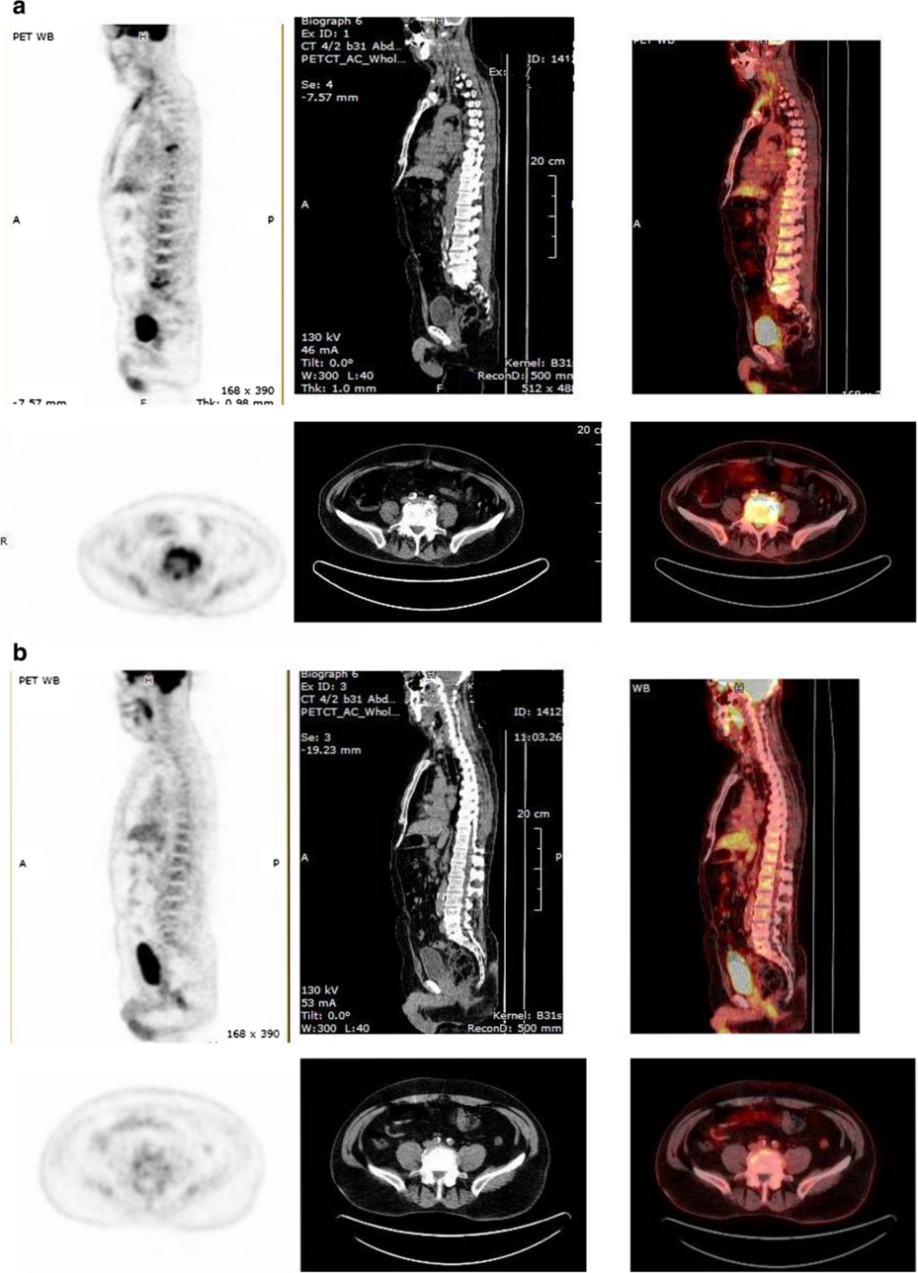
A. Sagittal (top) and coronal images of baseline PET/CT scan. Detailed legend: These images of patient no 4 show abnormal hypermetabolism at the left acromioclavicular joint, and at T6 and L5 vertebrae, along with paravertebral soft tissue involvement. Initial MRI evaluation of the lumbar spine of the same patient revealed only the lesion at the area of L4 – L5 vertebrae with paravertebral mass. B. Follow-up PET/CT scan after treatment. Detailed legend: These images of patient no 4 post-treatment demonstrate decreased FDG uptake at T6 and L5 vertebrae, and no uptake at the paravertebral tissues.
